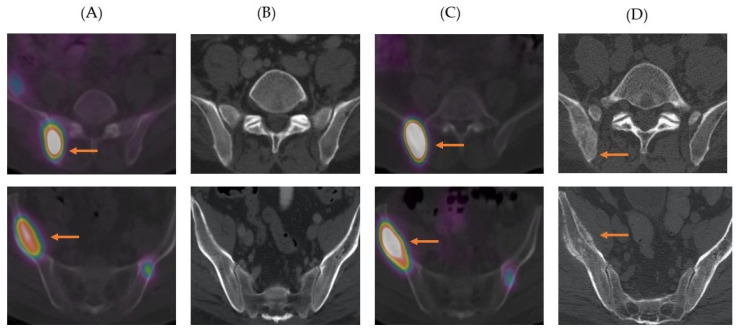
Primary pancreatic neuroendocrine tumor with metastases to liver, lymph nodes and bones. (A). Selected axial ^177^Lu-DOTATATE SPECT/CT image post first cycle of therapy shows multi-focal uptake in iliac bones. (B). Selected axial CT image before therapy shows no corresponding osseous lesion. (C). Selected axial ^177^Lu-DOTATATE SPECT/CT post fourth cycle of therapy shows persistent uptake at the similar locations. (D). Selected axial CT image after fourth cycle of therapy demonstrates sclerotic changes in the right iliac bone posteriorly and pathologic fracture and underlying sclerotic changes in the wing of right iliac bone.
